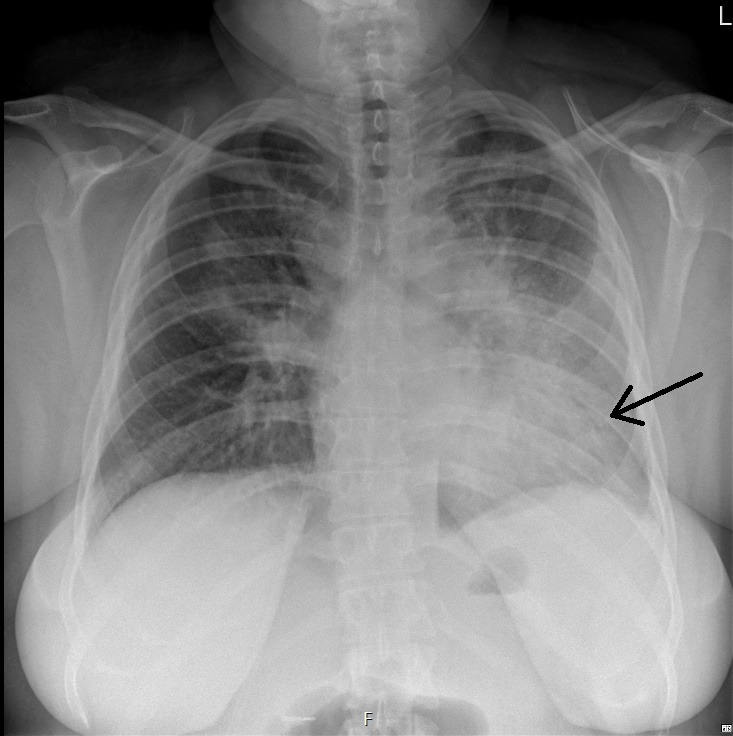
Posterior-anterior chest X-ray made on day 5 of admission showing consolidations in the left lobe (indicated by the arrow).
